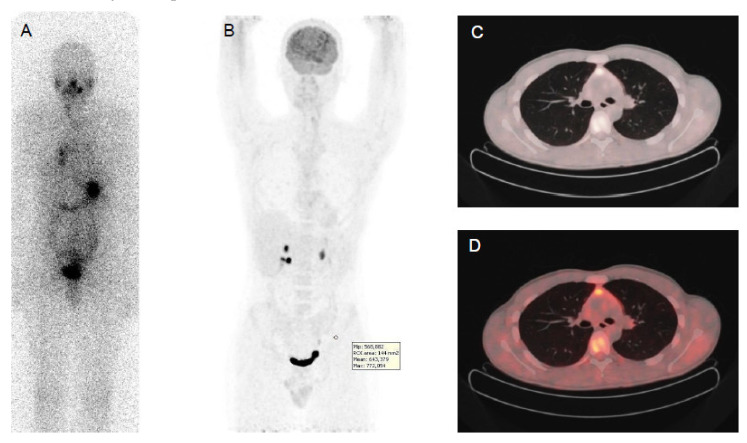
Papillary thyroid cancer in a 41-year-old man with a high thyroid stimulating hormone stimulated serum thyroglobulin level (475 ng/mL). Post-therapy (A) ^131^I whole-body scan showed pulmonary uptake and uptake and in neck lymph nodes. ^18^F-FDG PET-CT (B,C,D): CT images evidenced multiple small millimetric lung metastases (B,C,D), without significant ^18^F-FDG uptake.
